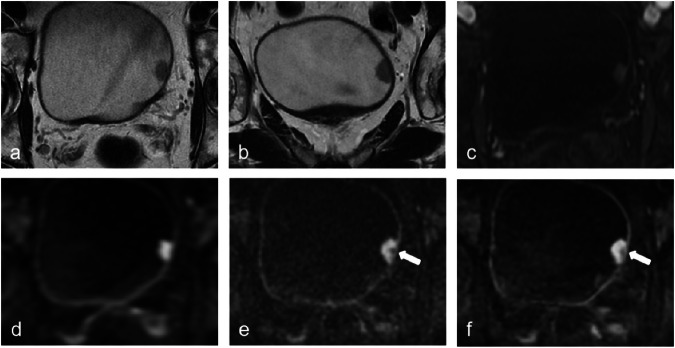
A 69-year-old man with pTa low-grade urothelial carcinoma. Axial (a) and coronal (b) T2WI show an exophytic tumor on the left side of bladder wall with no clear interruption of low-signal intensity muscularis propria, which was rated as T2 weighted category 3. DCEI (c) shows early enhancement of tumor, which was rated as DCEI category 3. fFOV DWI (d) shows a high signal-intensity tumor, which was rated as category 3. rFOV_STA_ DWI (e) and rFOV_DLR_ DWI (f) show high signal-intensity tumor with a low-signal-intensity stalk (arrow), which were rated as category 2. The VI-RADS score for set 1 was 3 for all readers, and the VI-RADS score for set 2 and set 3 was 2. DCEI, dynamic contrast-enhanced imaging; DWI, diffusion-weighted imaging; fFOV, full field-of-view; rFOV, reduced field-of-view; rFOV_DLR_, rFOV with DLR; T2WI, T2-weighted imaging
